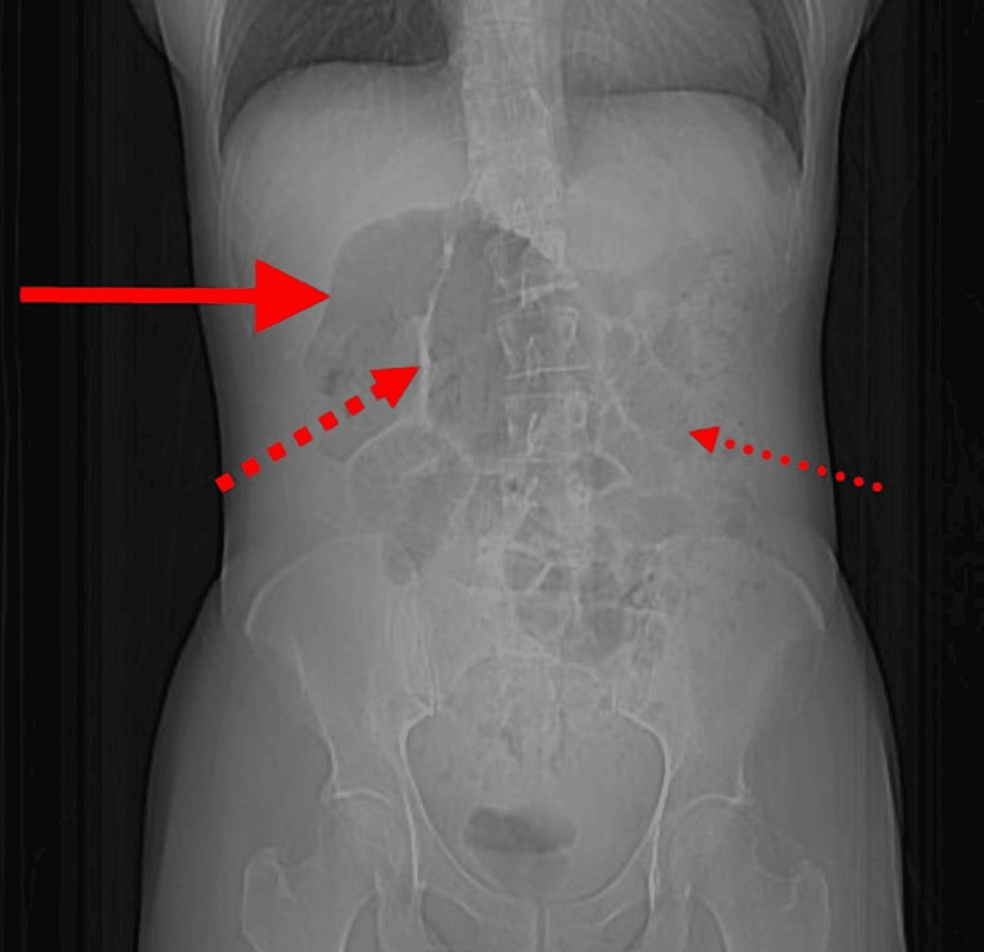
Scout CT scan image showing coffee bean sign (solid red line), split-wall sign (squared dot line), and oedematous bowel wall (round dot line).
